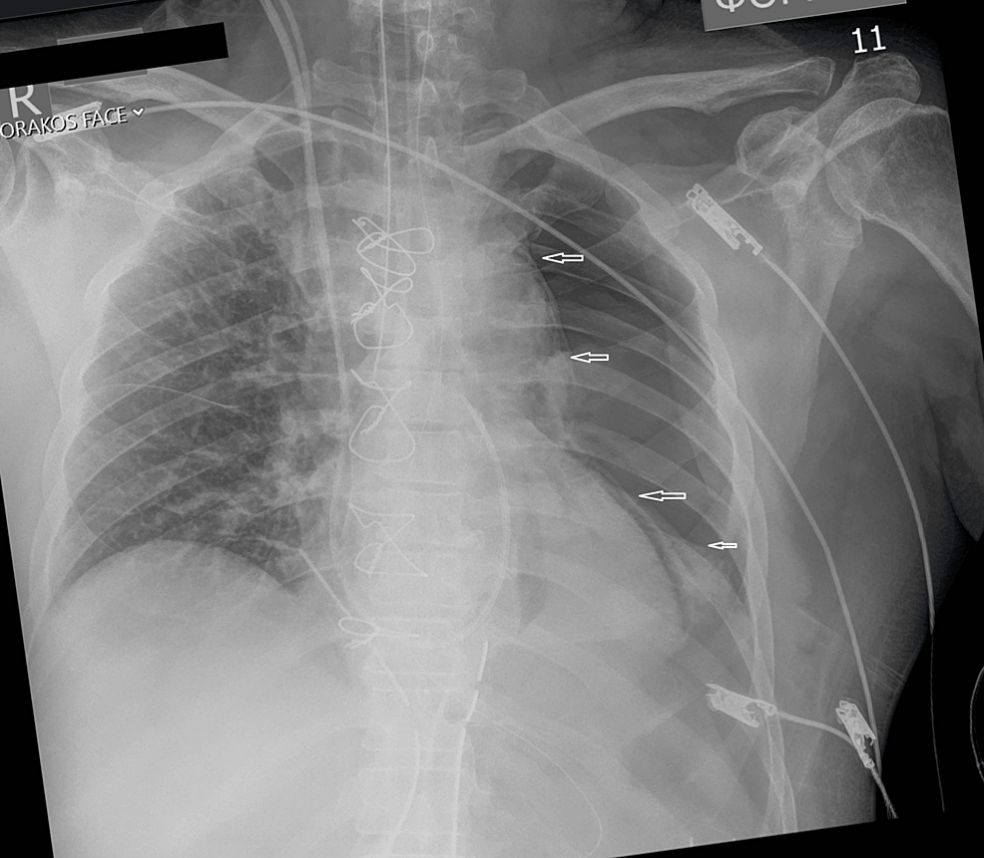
Initial portable postoperative chest radiograph showing total left pneumothorax. The arrows indicate the line of pneumothorax.
